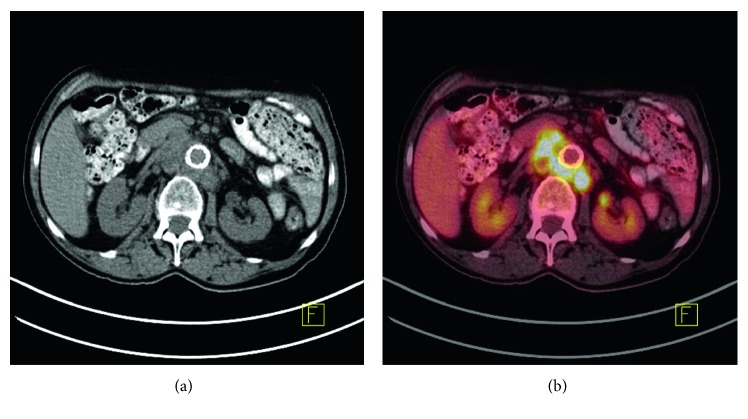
Axial CT and fused FDG PET-CT imaging demonstrating FDG-avid retroperitoneal lymphadenopathy displacing the abdominal aorta anteriorly.
